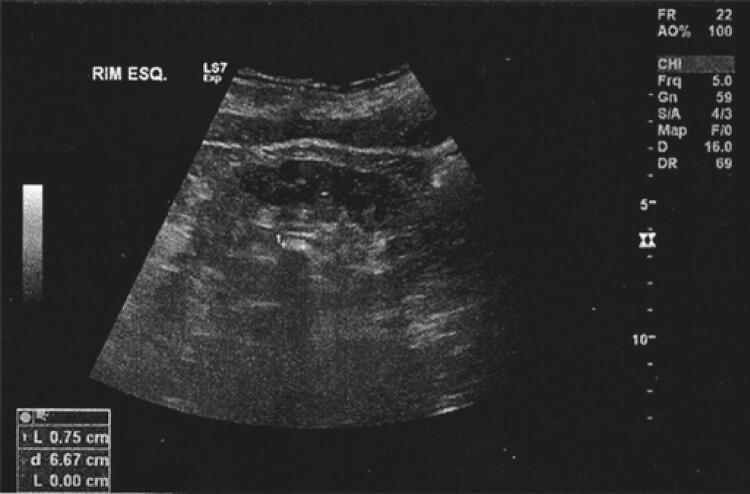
Ultrasonography suggestive of renal calculus of approximately 7.5mm in the middle third of the left kidney
